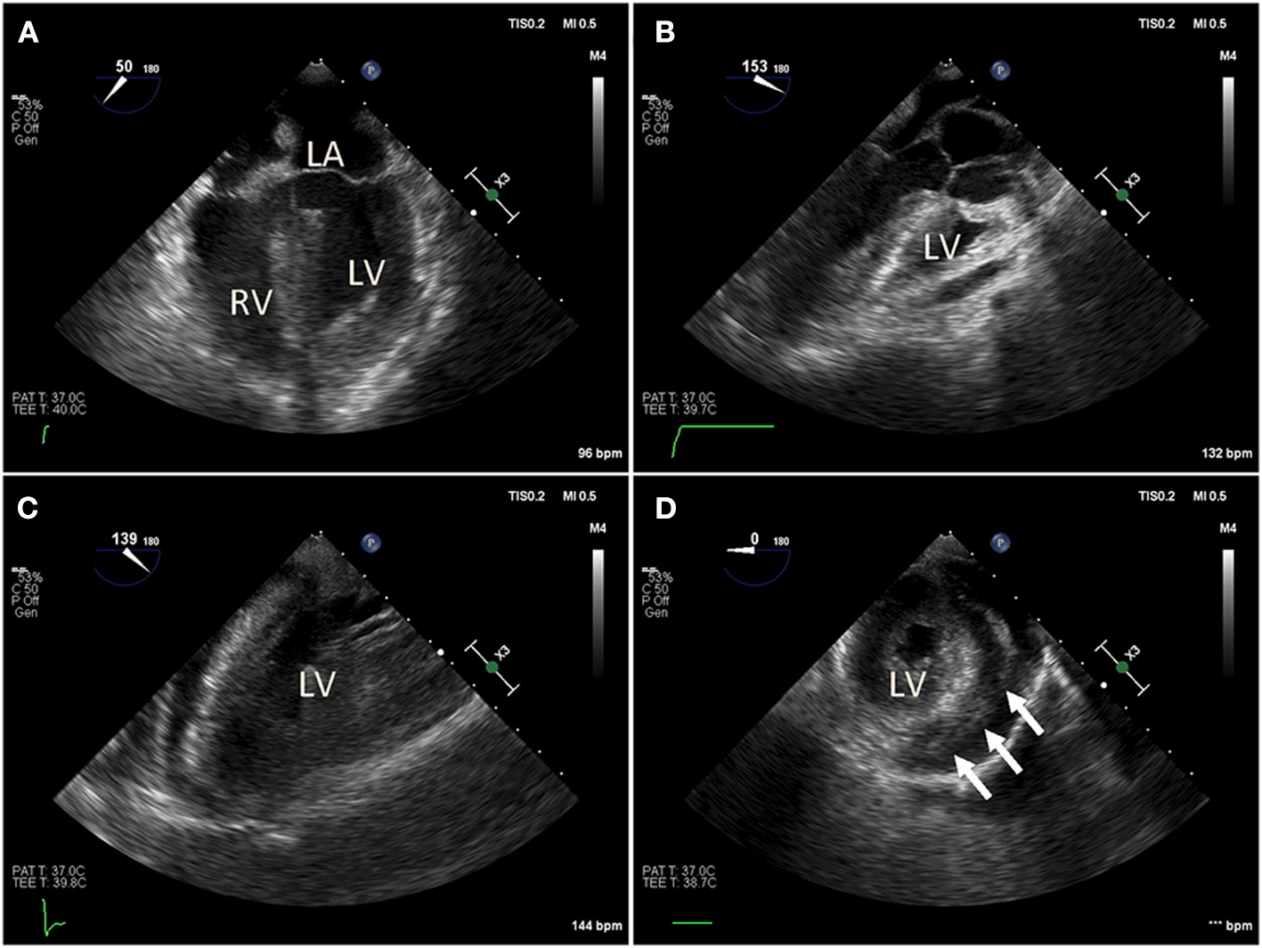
Intraoperative trans-esophageal echocardiography (TEE) in a COVID-19 positive patient with myocarditis, acute pericardial effusion and cardiac tamponade. After bedside venous-arterial extracorporeal membrane oxygenation (VA-ECMO) cannulation was performed, the patient was taken to the operating room emergently for ventral cardiac window exposure and decompression of the pericardial effusion. (A) Preoperative mid-esophageal four chamber view demonstrated severely reduced global left ventricle (LV) function. (B,C) Similarly, mid-esophageal long axis view demonstrated severely reduced LV contraction. (D) Transgastric short axis view demonstrated under-filling of the LV. Patient was taken emergently to the operating room for cardiac window decompression of the pericardial effusion and a large pericardial effusion. An Impella device is seen in the LV.
